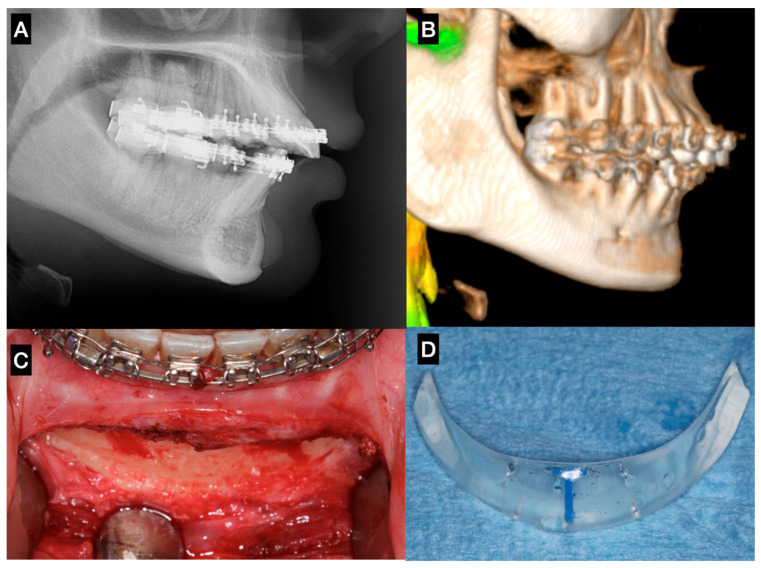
Sagittal chin augmentation using a standard silicone implant in a patient presenting with a Class II skeletal deformity. The patient sought evaluation due to implant mobility detected clinically four years after placement. (A) Lateral cephalometric radiograph showing pronounced bone resorption beneath the implant, with proximity to the apices of the mandibular incisors. (B) 3D reconstruction confirming a well-defined resorption defect extending toward the mental foramen. (C) Post-explantation intraoperative image demonstrating the full extent and depth of the osseous defect. (D) Removed standard silicone implant, evidencing its non-anatomic contour and excessive posterior extension, factors that likely contributed to instability and bone resorption—Image from the authors’ personal archive.
